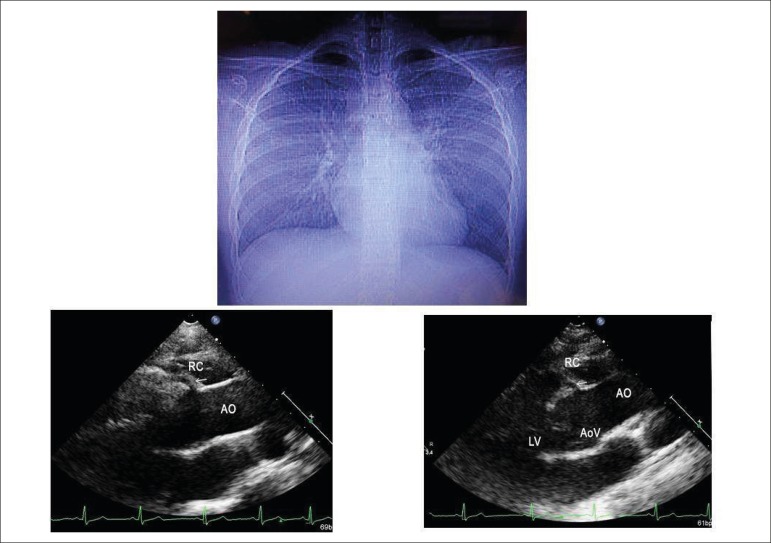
Chest X-ray shows normal cardiac area and pulmonary vascular markings
